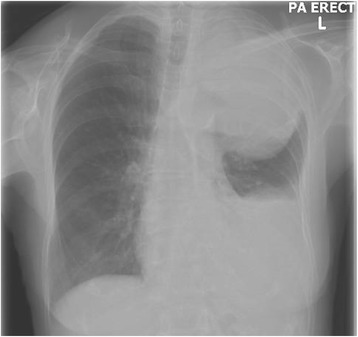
Chest radiograph showed a huge mass in the upper left hemithorax and an ipsilateral moderate-sized pleural effusion
